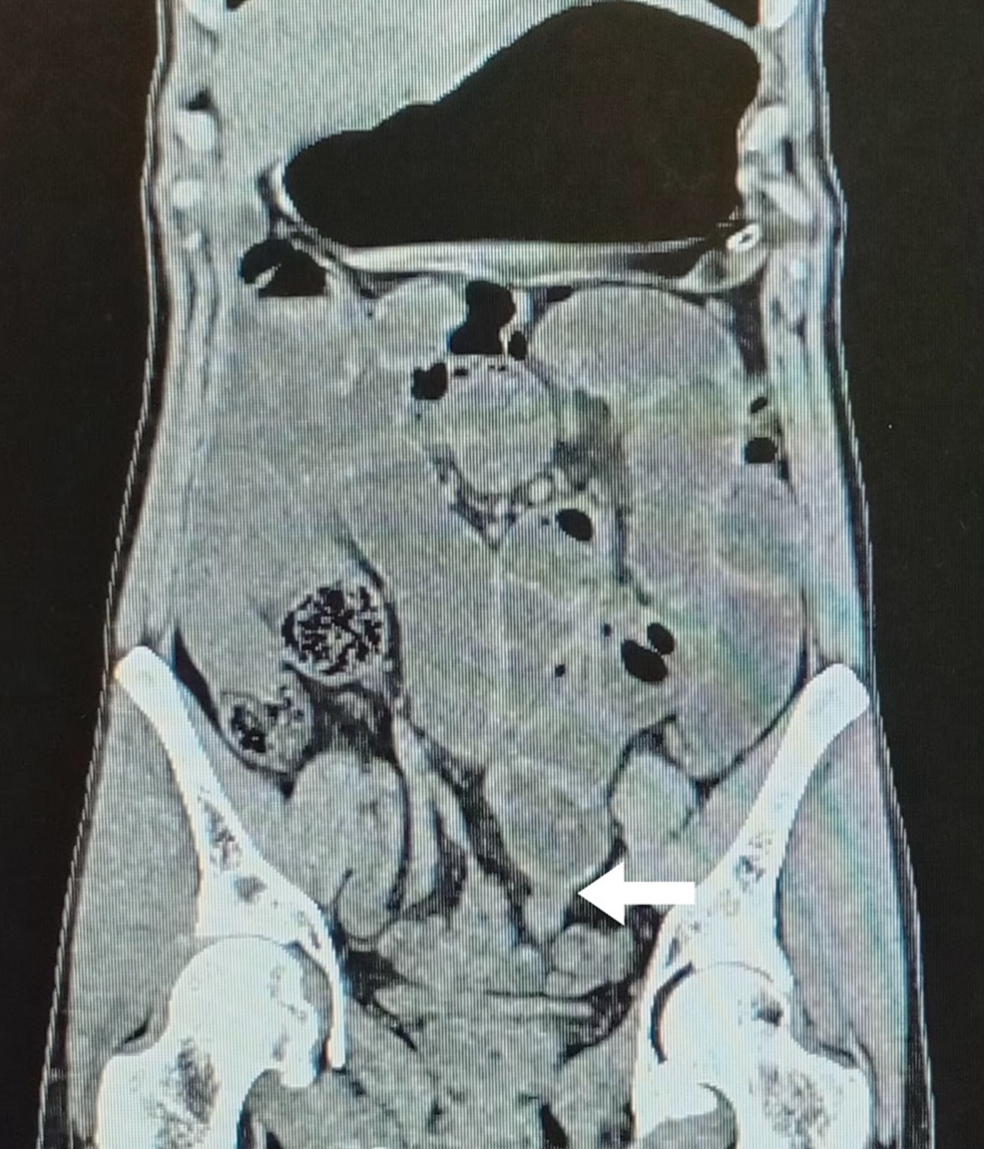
Non-contrast computed tomography of the abdomen showing multiple dilated small bowel loops with the zone of transition in the terminal ileum (white arrow). The appendix could not be identified separately from the ileal loops.
